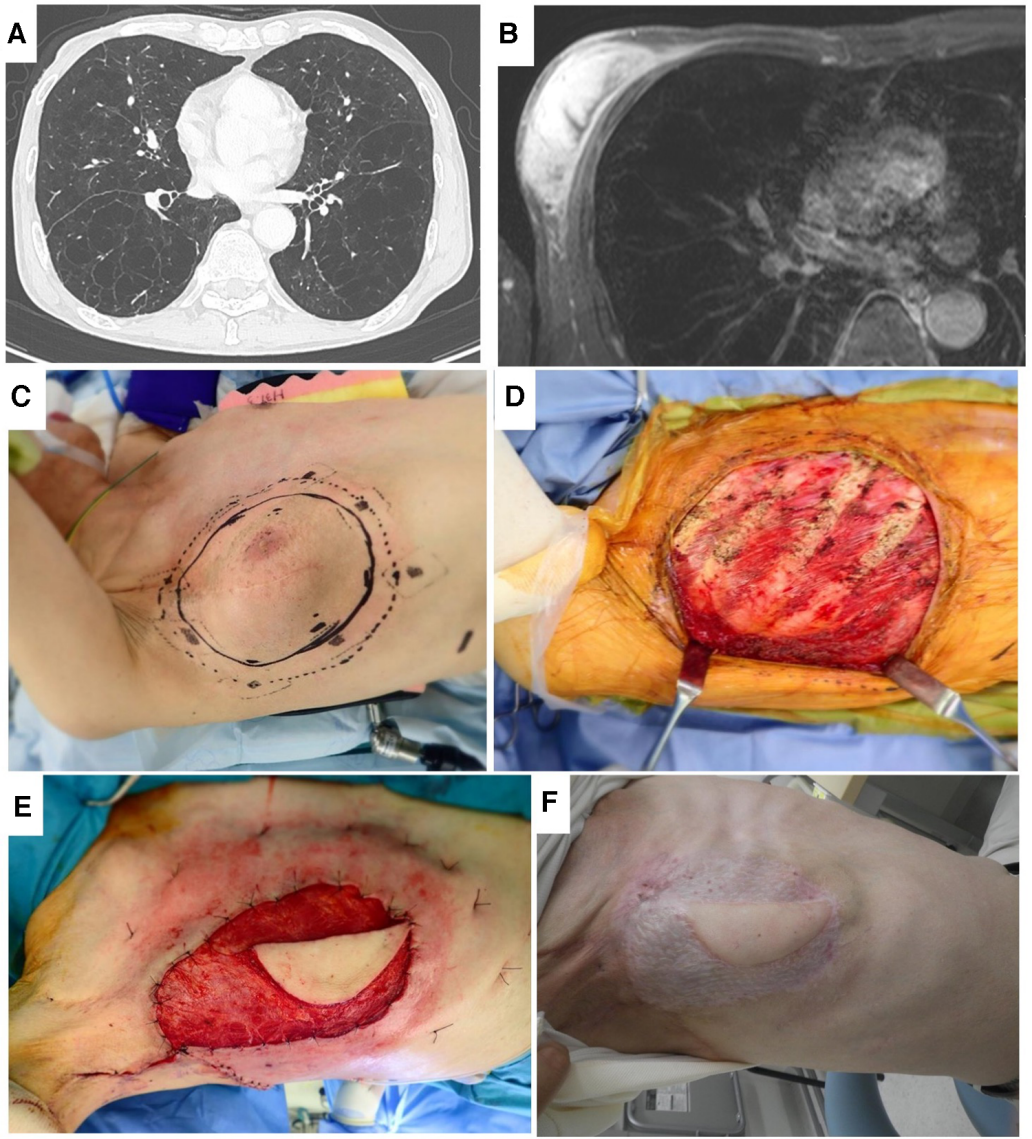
Patient No. 3. Lung CT (A), axial MRI (B), resection area (C), after resection (D), coverage by same-side LD before skin graft (E), and healed by skin graft (F).
